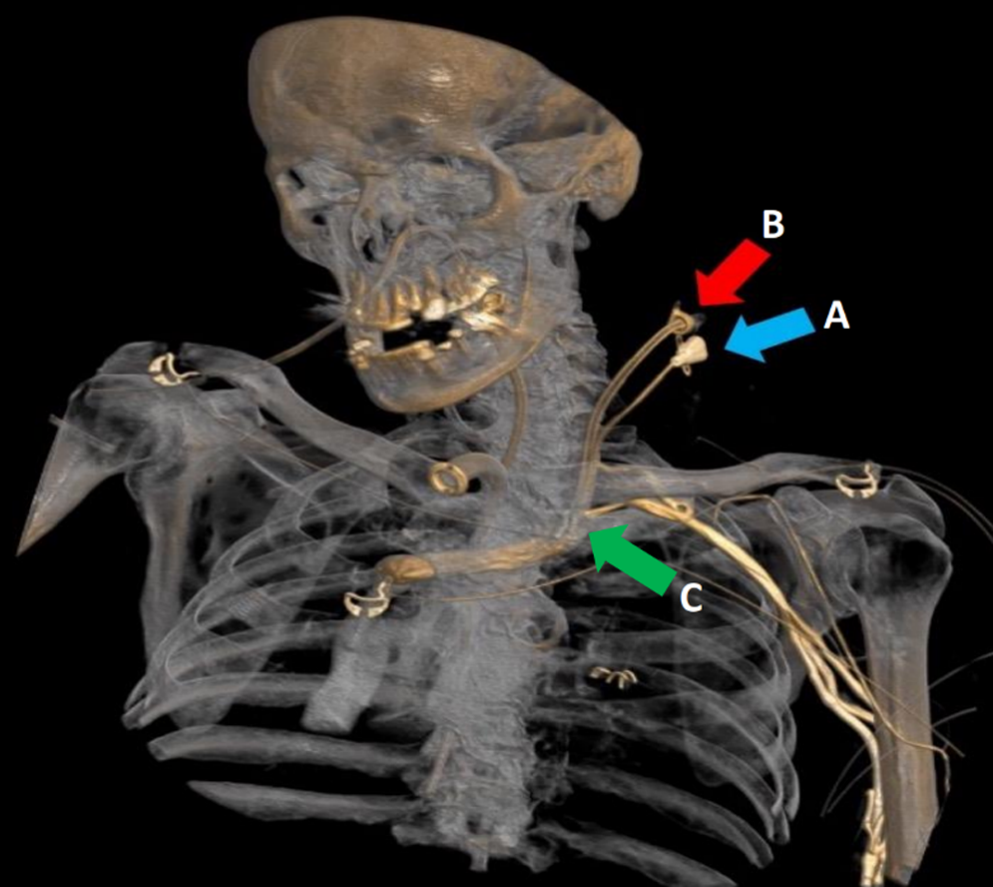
Reconstructed 3D image of CT angiogram of the aortic arch and carotid arteries. The CVC (A) and dialysis catheter (B) can be seen entering the left aspect of the neck and the left internal jugular vein. The CT report confirmed the presence of both catheter tips in the distal left brachiocephalic vein (C). Contrast is noted to obscure the distal part of both catheters in this image.
